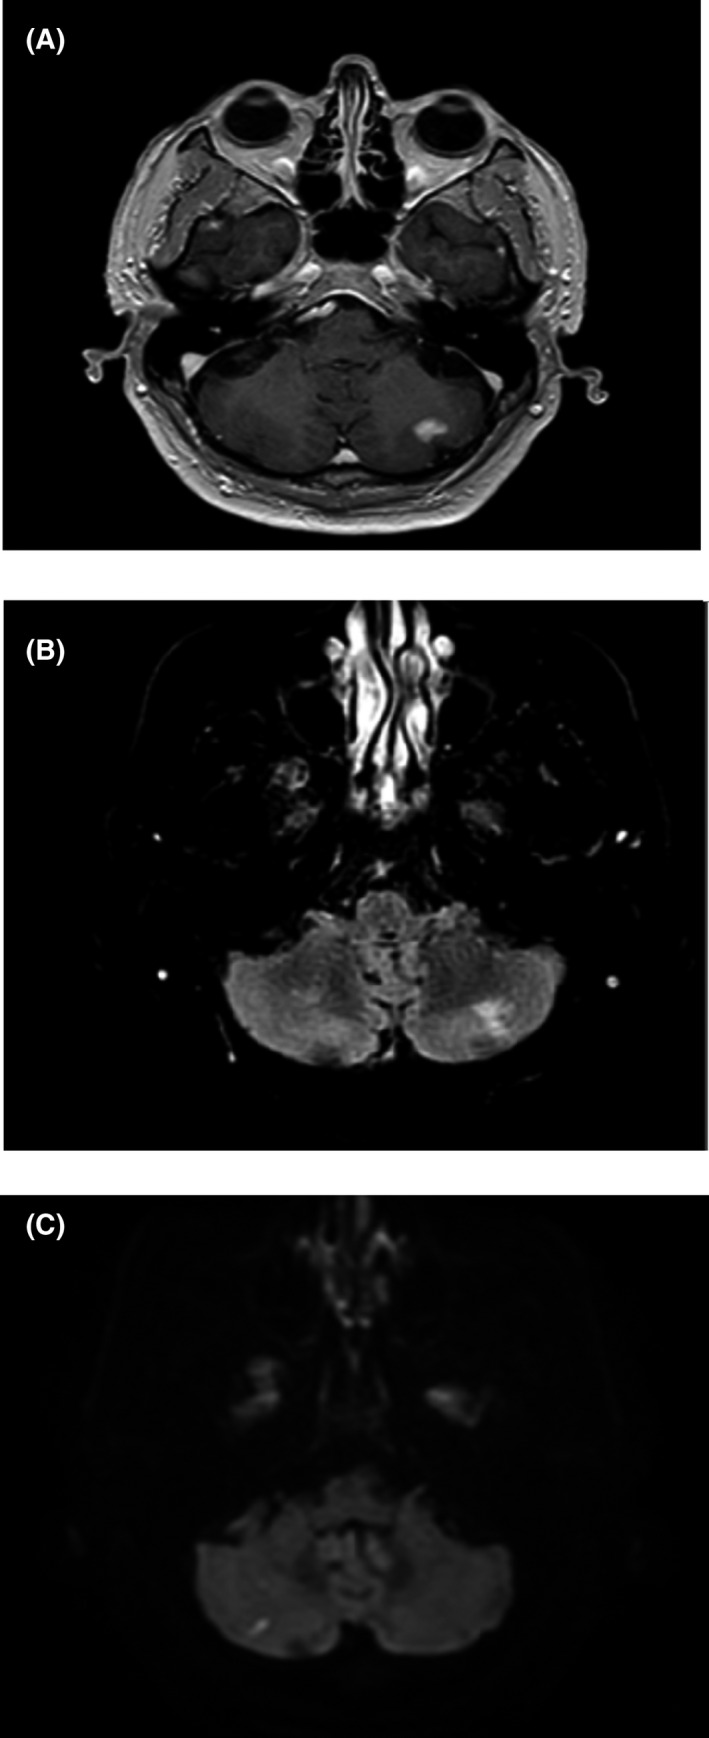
Magnetic resonance (MR) imaging of the brain revealed a small, acute right cerebellar infarct, subacute left cerebellar infarct, and mild bifrontal chronic microvascular ischemic changes (A = T1 sequence, B = T2 FLAIR sequence, and C = DWI sequence)
